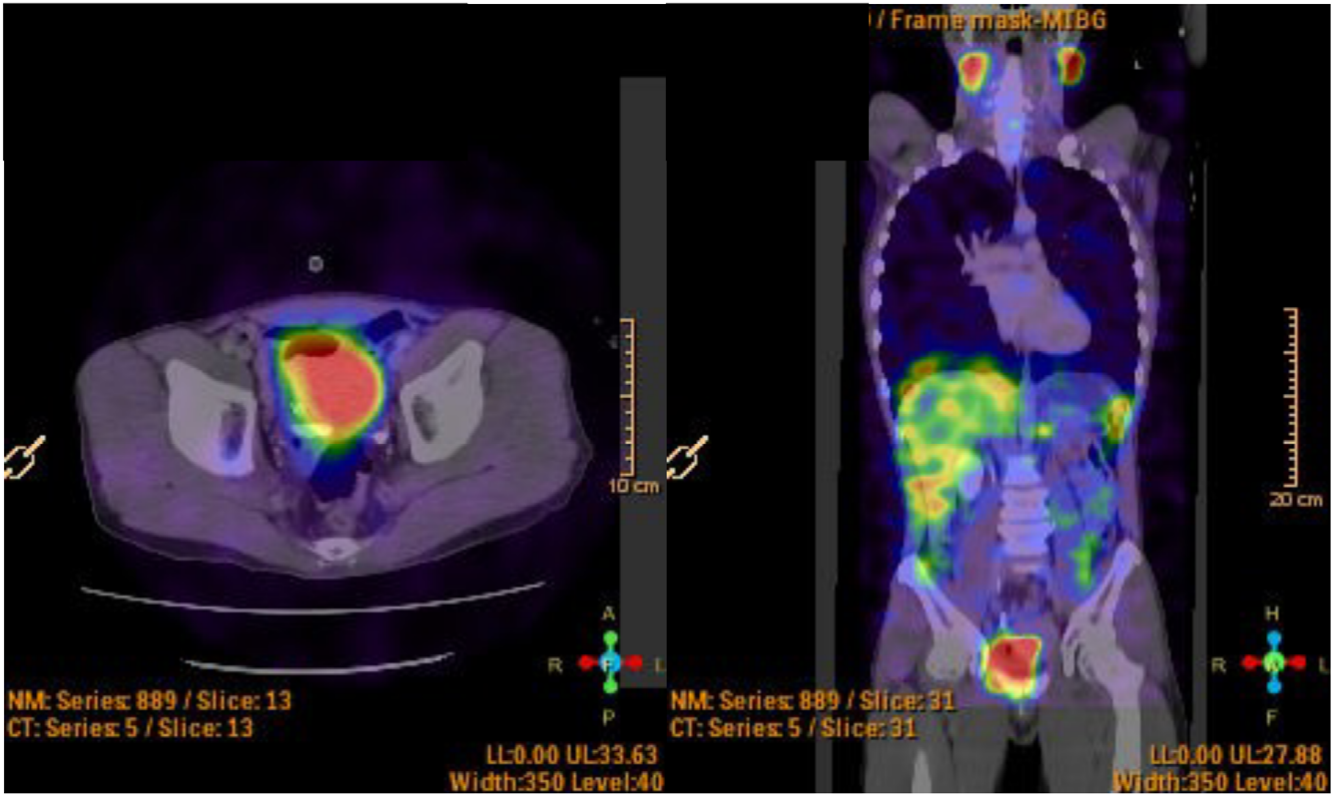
The ^123^I‐MIBG scintigraphy revealed accumulation consistent with a bladder mass.
